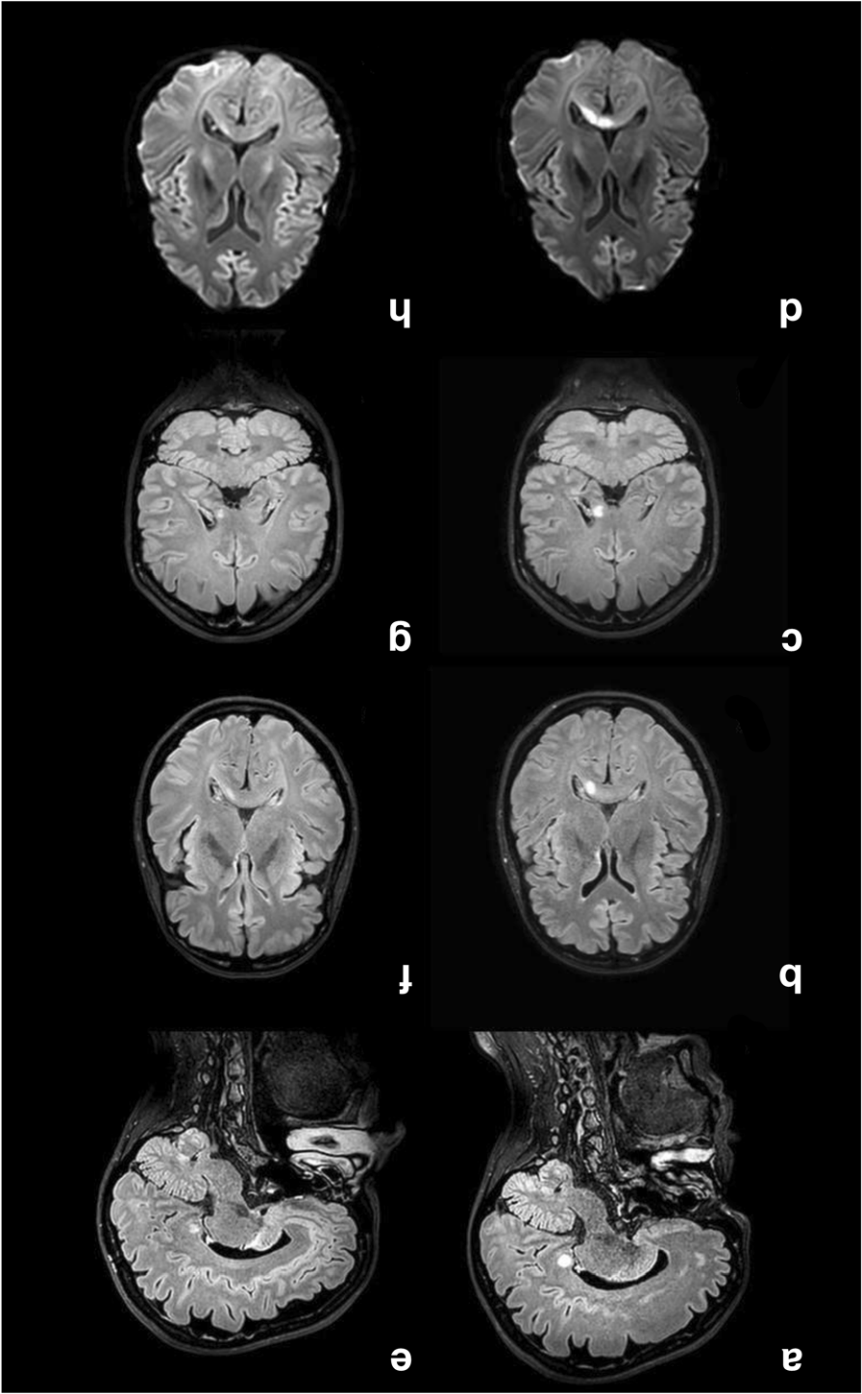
Neuroradiological evolution of the splenial lesion at repeated brain MRI scans, performed during hospitalization (a-d) and 6 months after the clinical onset (e-h). Fluid-attenuated inversion recovery sequences on a sagittal section (a, e), on a coronal one (b, f) and on an axial plane (c, g). Diffusion restricted images (d, h) show a resolution of the cytotoxic edema on follow-up MRI scans
